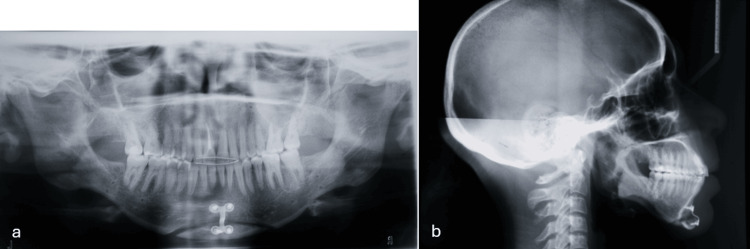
Post-treatment records. (a) Panoramic radiograph. (b) Lateral cephalometric radiograph.
